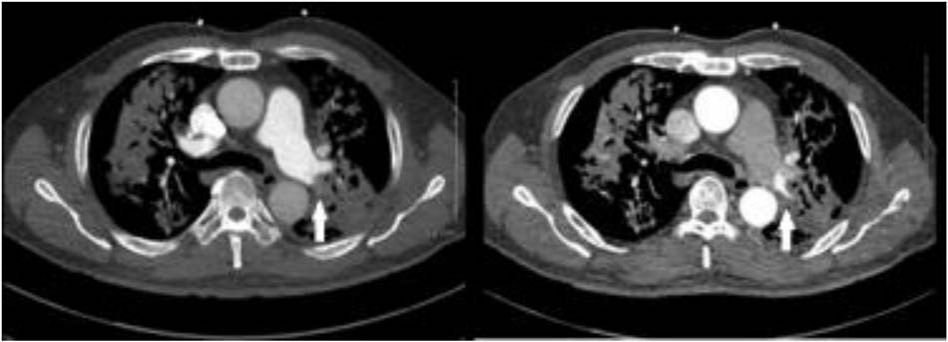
CT pulmonary angiogram (left) demonstrates non-opacifying segmental pulmonary artery (white arrow); on the CT systemic arterial phase angiogram (right), the same segmental pulmonary artery opacifies; background cavitating consolidation is seen in both lungs.
